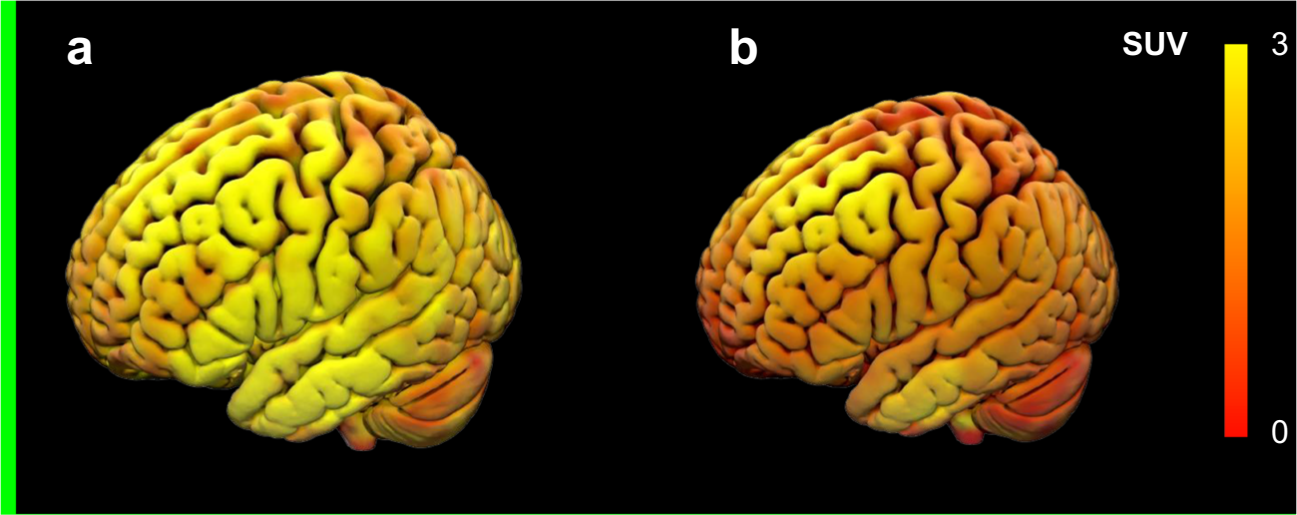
[11C]PBR28 brain PET tissue uptake (quantified with SUV) before (A) and after XBD173 administration (B). Images taken from scans of the same representative patient to show the effect of XBD173 on tracer uptake across the brain
